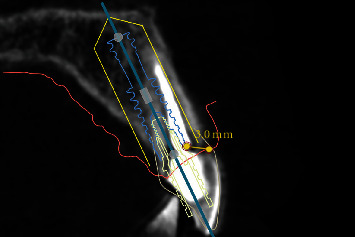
Sagittal view of the CBCT scan showing the prosthetically and biologically driven implant planning.
